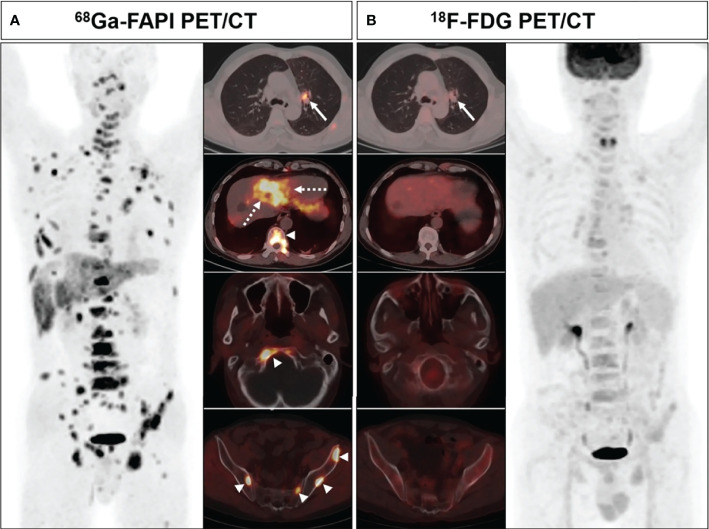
A 63-year-old male (patient 18) diagnosed with adenocarcinoma. ^68^Ga-FAPI PET/CT (A) showed intense tracer uptake in the primary tumor (solid arrows, SUVmax=10.0), liver metastasis (dashed arrows, SUVmax=7.6) and bone metastases (arrows, SUVmax=8.3-8.5). ^18^F-FDG PET/CT (B) showed primary lesion with mild FDG uptake (solid arrows, SUVmax=3.6), while no significant FDG uptake was showed in liver metastasis and multiple bone metastases.
